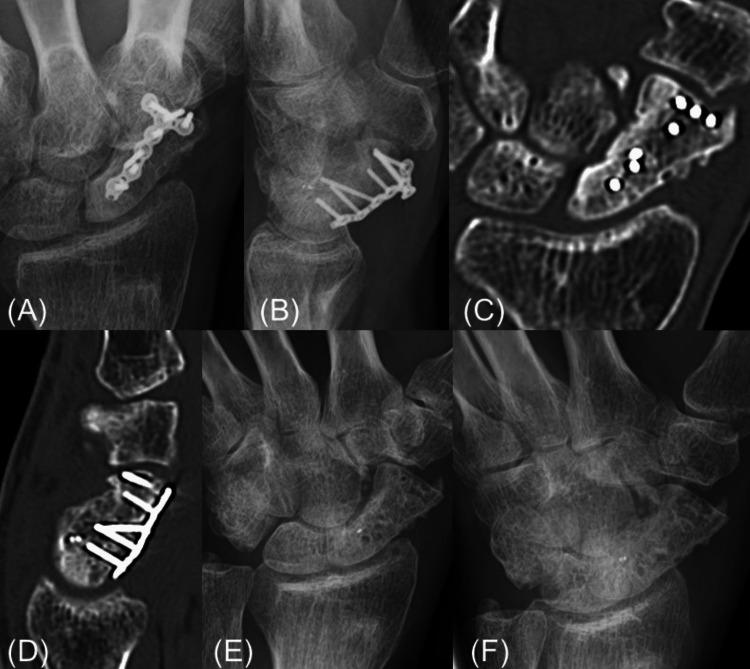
Radiographs and CT scans before and after locking plate removal. Radiographs and CT scans before and after locking plate removal, confirming union. (A, B) The radiographs show the scaphoid union. (C, D) The CT images similarly confirm the union. (E, F) The radiographs after the locking plate removal show the scaphoid union.
